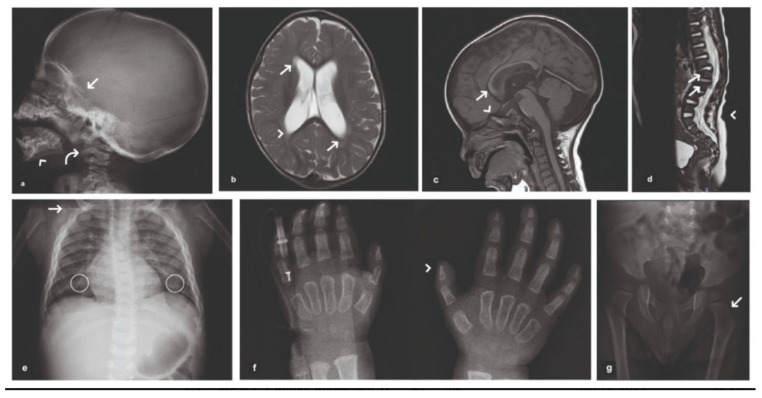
Index patient’s X-ray images. (a) Lateral skull X-ray: macrocephaly with abnormal J-shaped sella turcica (arrow); molars unerupted and angulated in both jaws (arrowhead); C2 odontoid process hypoplasia (curved arrow). (b) MRI T2: patched hyperintensity areas (arrows); Evans’ index of 0.3; fourth ventricle dilatation (arrowhead). (c) MRI T1: thin corpus callosum (arrow); sella turcica dysplasia (arrowhead). (d) MRI T2: L1-L2 dysplasia (arrow); exuberant kyphotic curve (arrowhead). (e) Anterior posterior chest X-ray: perihilar infiltrate and peribronchial thickening (circles). (f) Anterior posterior hand plate X-ray: cone-shaped phalanges (arrowhead). (g) Pelvic X-ray: bilateral coxa vara (arrow).
